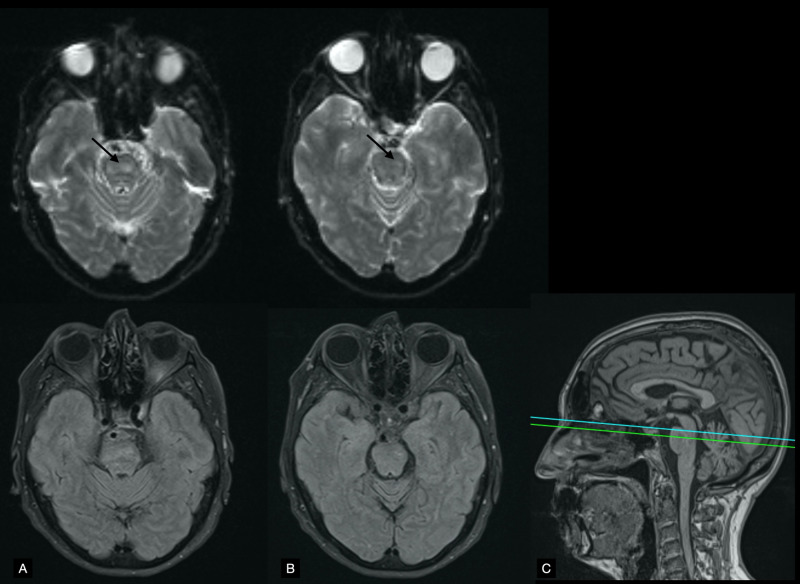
Brainstem MRI Panel A shows axial diffusion-weighted imaging (DWI) of the mid pons (top) and the corresponding fluid-attenuated inversion recovery (FLAIR) image (bottom). Panel B shows axial DWI of the rostral pons (top) with the FLAIR image (bottom). Notice the presence of matching hyperintense lesions on DWI and FLAIR (black arrows), suggesting they are older than 4.5 hours. Panel C shows the positions of A (inferior, green line) and B (superior, blue line) relative to a T1-weighted image of the midline sagittal plane. Apparent diffusion coefficient (ADC) values were decreased.
